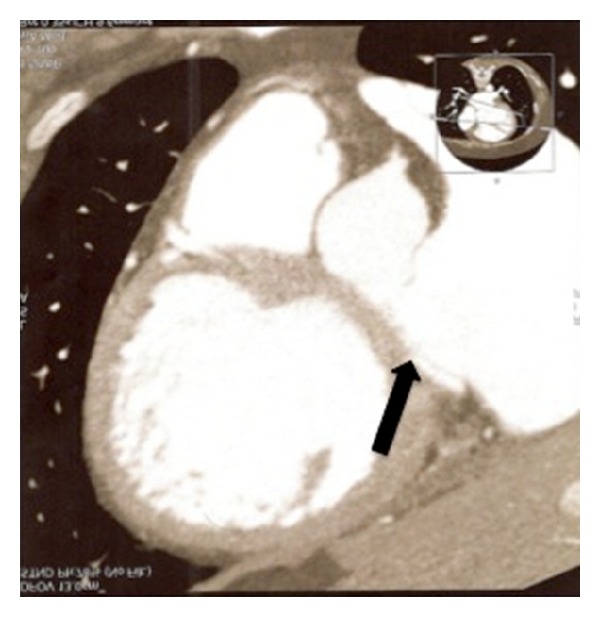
Cardiac CT demonstrating aortic right ventricular fistula.
